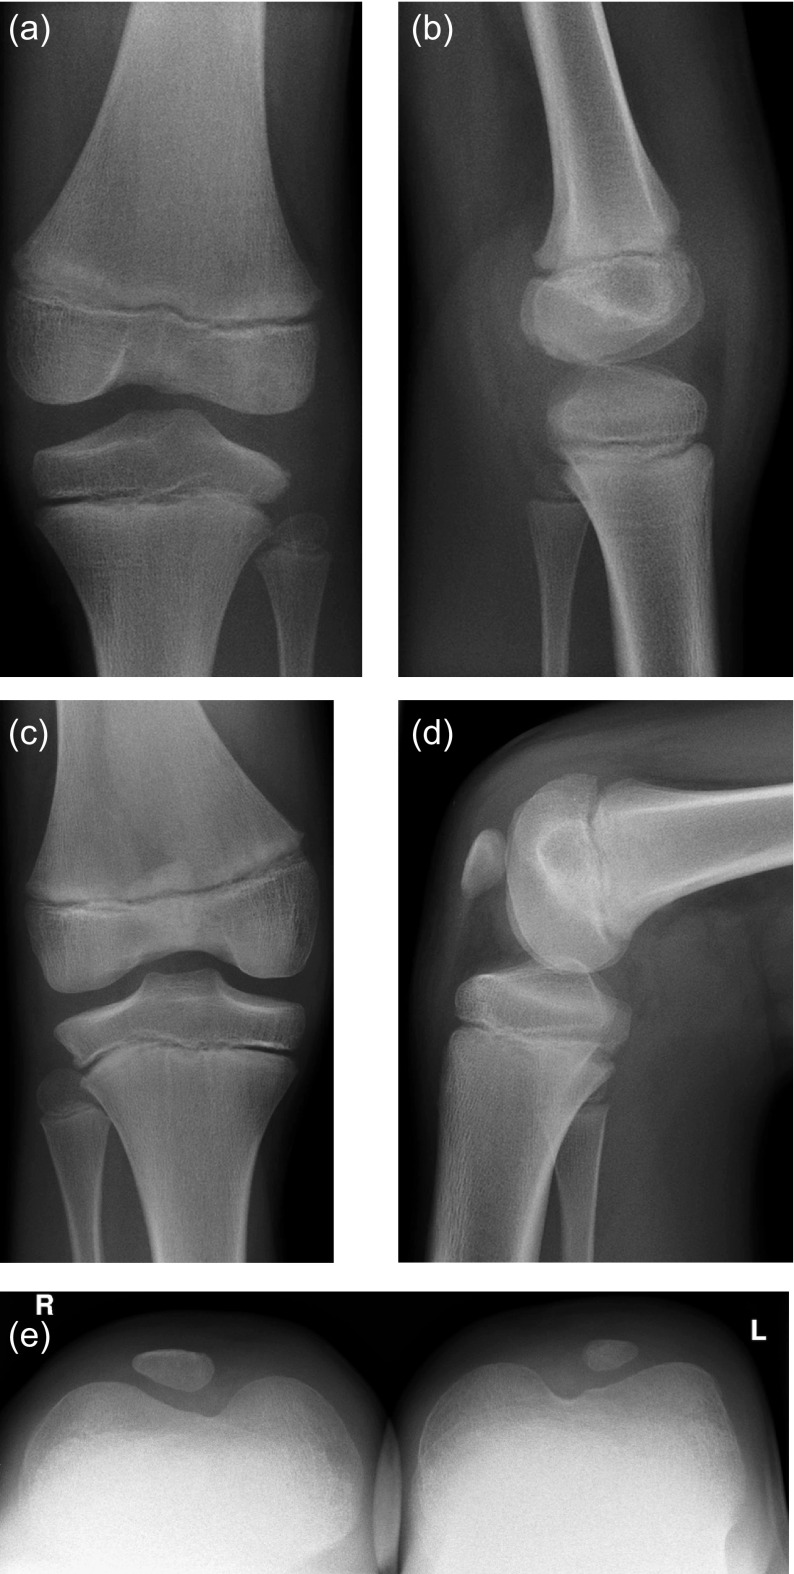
(a, b) Radiological follow-up two years postoperatively at age of eight no patella is seen on X-rays. (c–e) Six years postoperatively at age of 12 patellae are hypoplastic but centered and trochleae are deep.
